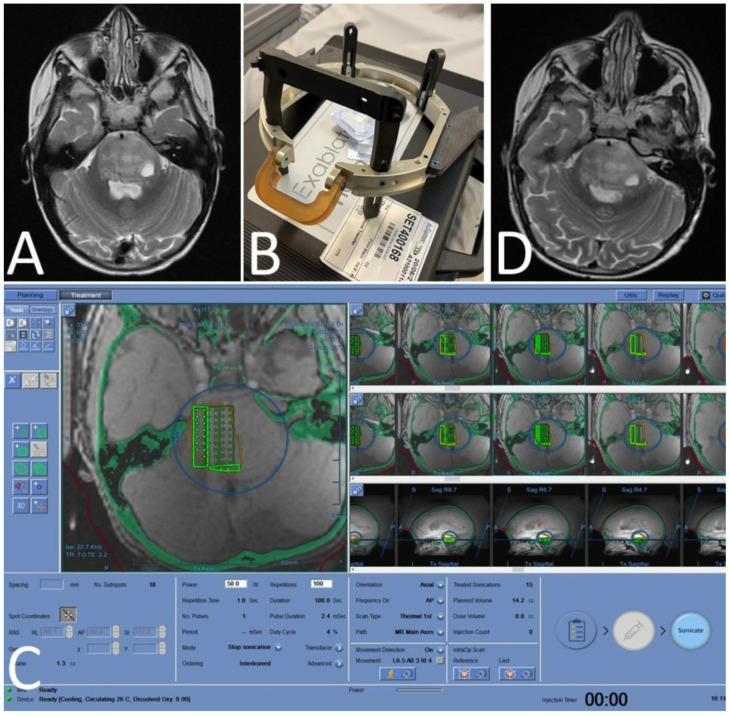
Sonodynamic therapy to the right half of the pons for DIPG in a 5-year old-child [29]. (A) pre-procedure MRI demonstrating diffusely infiltrating lesion in the pons; (B) focused ultrasound headframe; (C) planning station demonstrating sonication targets; (D) post-procedure MRI demonstrating no adverse changes.
